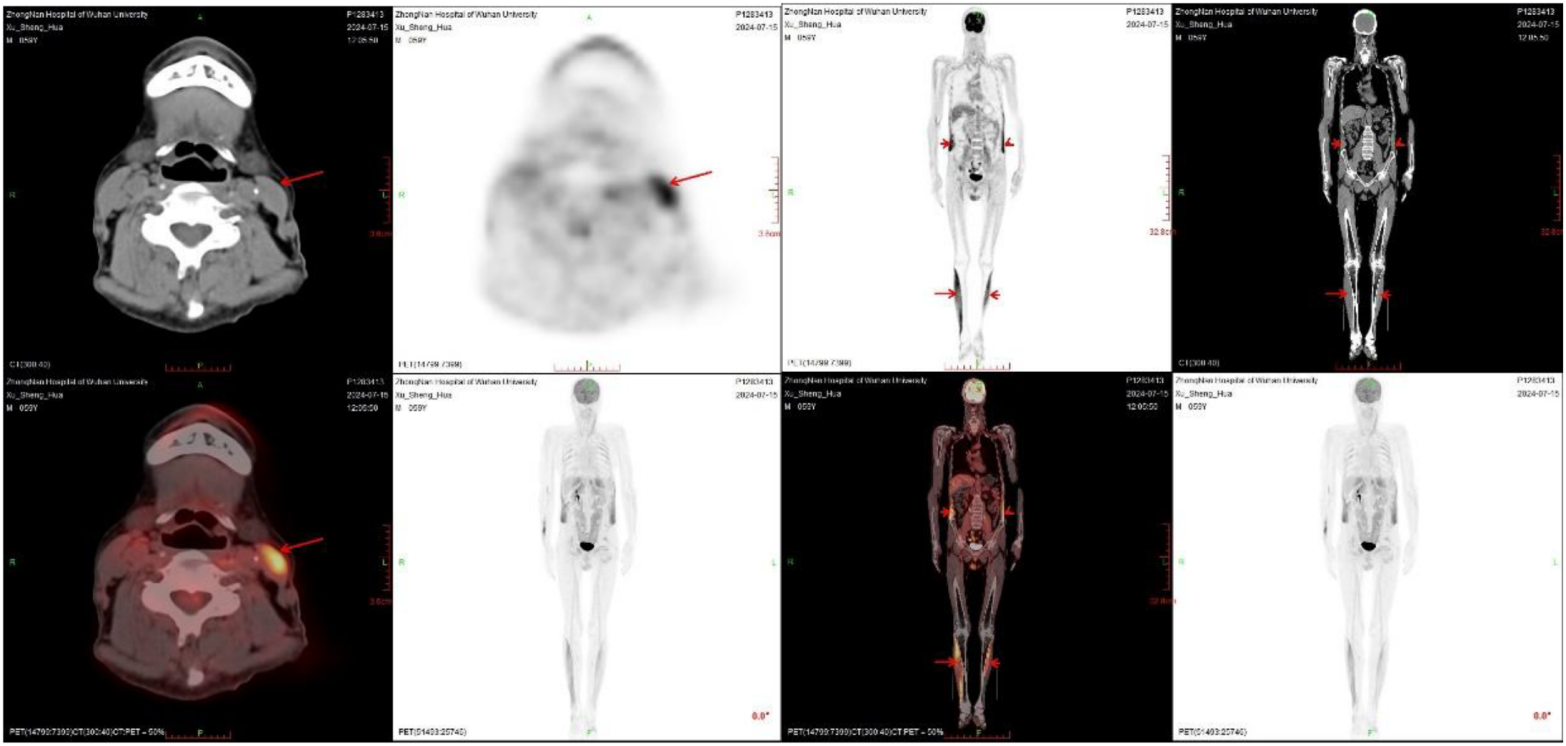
Whole-body PET–CT imaging in stiff-person syndrome. Symmetric increased ¹^8^F-fluorodeoxyglucose (FDG) uptake is seen in bilateral shoulder girdle muscles (standardized uptake value [SUV]_max_, 4.2), paraspinal muscles (SUV_max_, 3.6), and hip adductor muscles (SUV_max_, 3.9) (red arrows), reflecting sustained muscle contraction characteristic of stiff-person syndrome.
